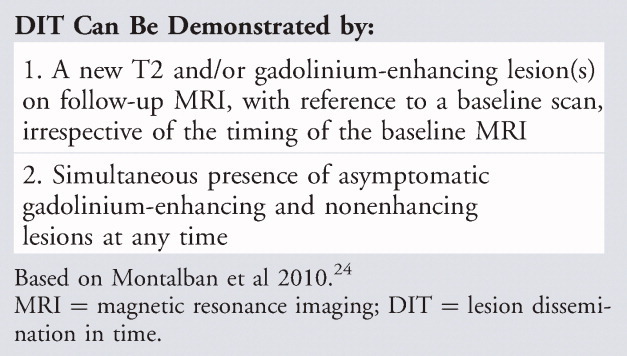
By using the recommended simplified MAGNIMS criteria to demonstrate DIS^22^ and allowing DIT to be demonstrated by a scan containing both enhancing and nonenhancing lesions in regions of the CNS typical for MS,^23^ a diagnosis of MS can be made in some CIS patients based on a single MRI.^24^ The Panel felt this is justified because it simplifies the diagnostic process without reducing accuracy. However, a new clinical event or serial imaging to show a new enhancing or T2 lesion will still be required to establish DIT in those patients who do not have both gadolinium-enhancing and nonenhancing lesions on their baseline MRI.
